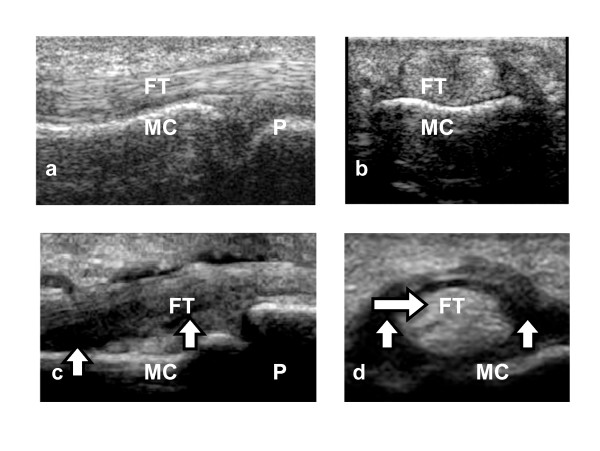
Ultrasound appearance of normal flexor tendon sheath and tenosynovitis. a, Normal appearance, longitudinal view. b, Normal appearance, transverse view. c, Flexor tenosynovitis, longitudinal view. d, Flexor tenosynovitis, transverse view. Arrows in c and d indicate tendon sheath thickening. MC: metacarpal; P: phalanx; FT: flexor tendon
